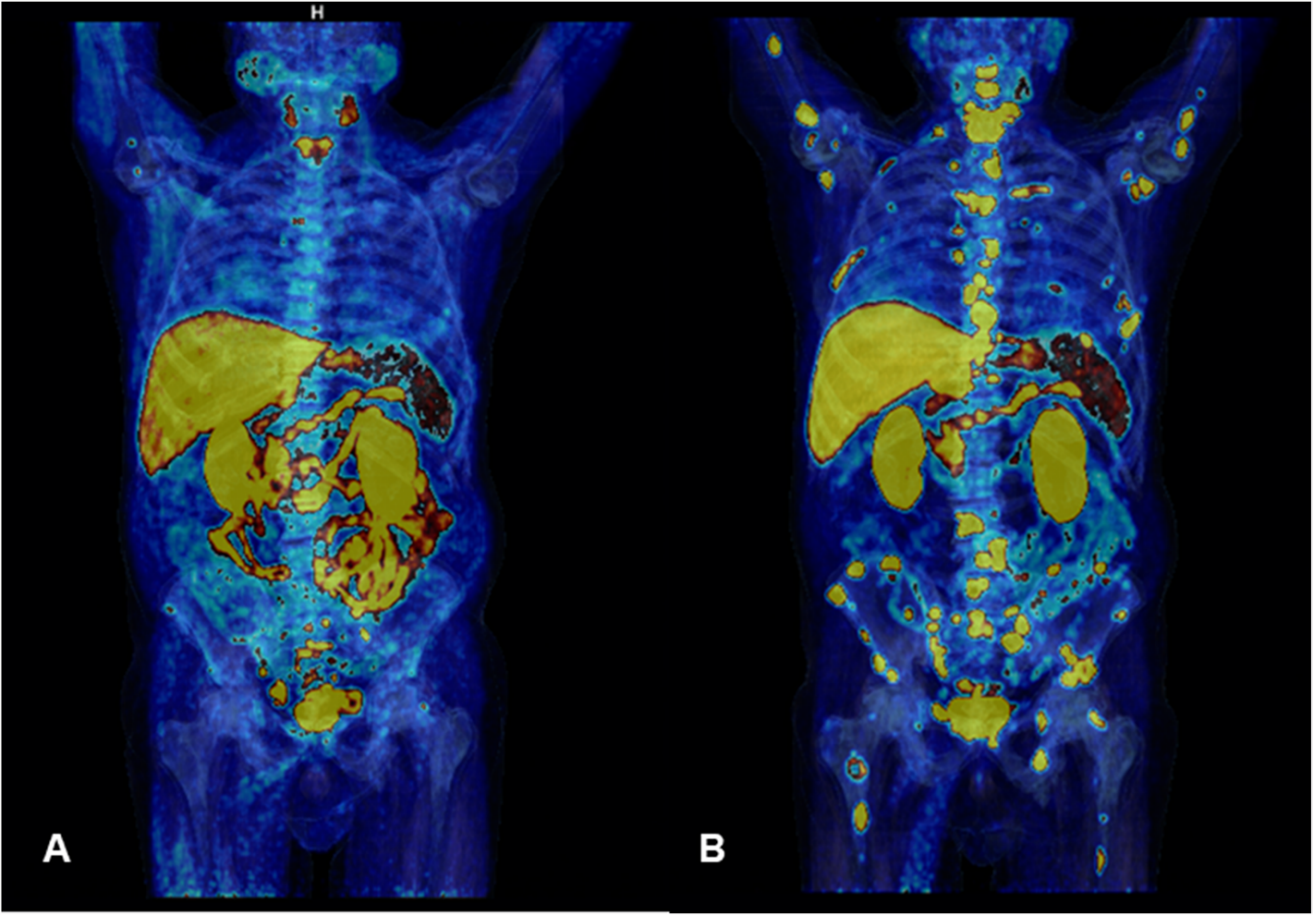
Baseline and post enzalutamide [18F]F-choline maximum intensity projection images (A and B respectively). Restaging [18F]F-choline PET/CT showed the appearance of multiple skeletal uptakes (i.e. SUVmax 21.3 on sternal body)
